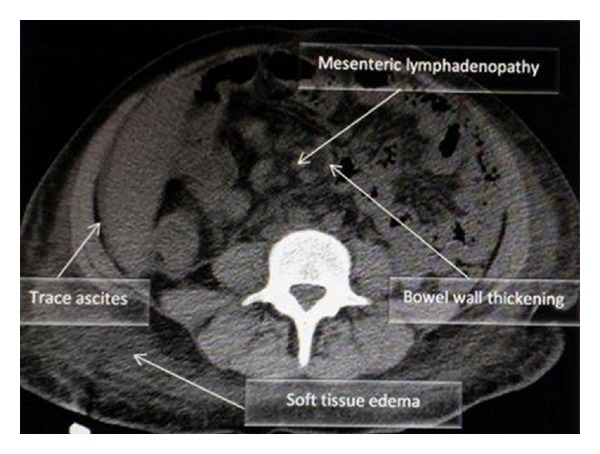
Abdominal CT scan which showed mesenteric lymphadenopathy, bowel wall thickening, trace ascites, and soft tissue edema.
